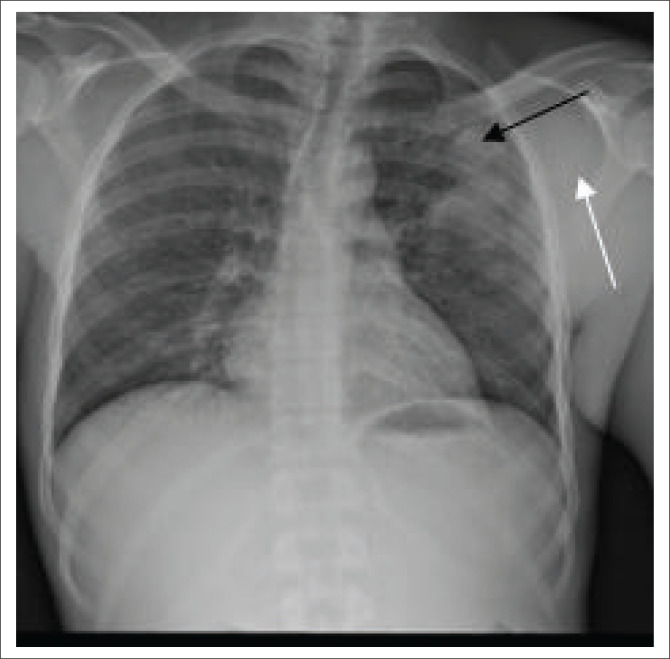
Frontal chest radiograph: left upper lung airspace opacity with internal cavitation (black arrow) and left axillary soft tissue swelling (white arrow).
